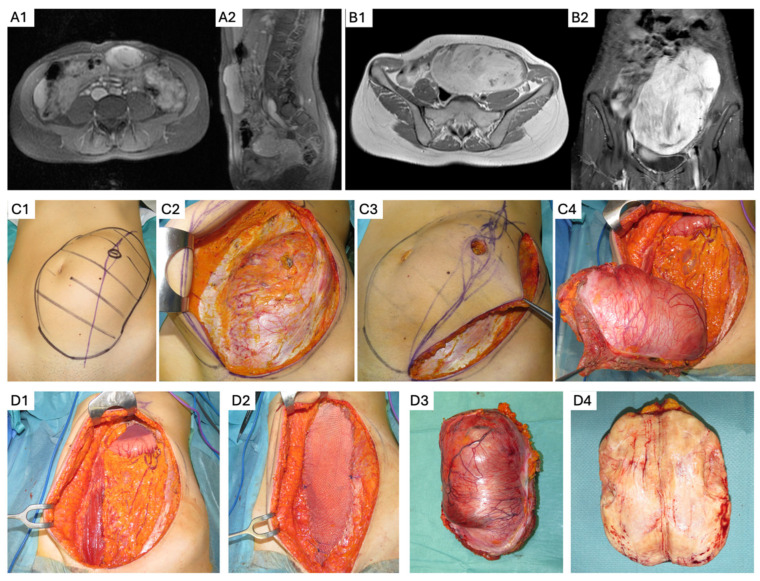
Surgical management of abdominal wall desmoid tumor. (A1,A2) Axial and sagittal T1 MRI of a sporadic lower abdominal wall desmoid in a 30-year-old female patient. (B1,B2) She had progressive growth and increasing symptoms, remaining on active surveillance for 2 years. (C1–C4) She underwent myofascial resection, including the biopsy tract, with narrow clinical margins and mesh reconstruction (D1,D2), with no complications. Pathology showed negative margins (D3,D4), and she underwent surveillance. All surgical images of this manuscript were retrospectively collected from routine clinical procedures and have not been published before. The use of these images is in accordance with the Declaration of Helsinki. The patient consent is waived because of the retrospective analysis, and all patient identifiers have been removed.
